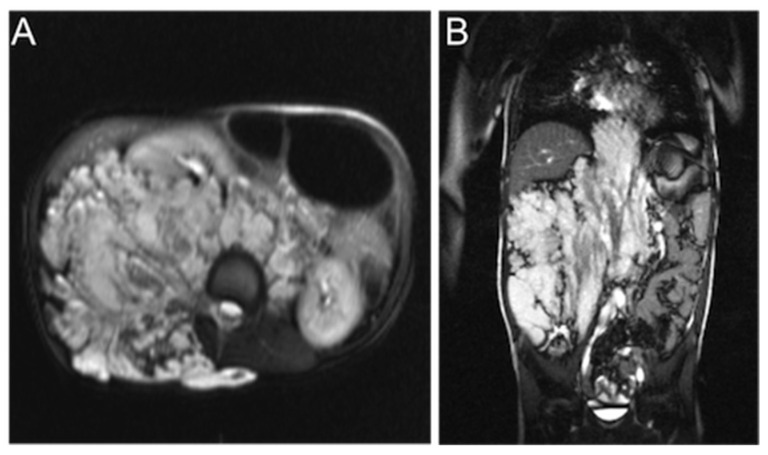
Axial (A) and coronal (B) T2-weighted MR images of an extensive VM affecting the retroperitoneum and spinal muscles and dislocating the bowel and the right kidney to the left.
